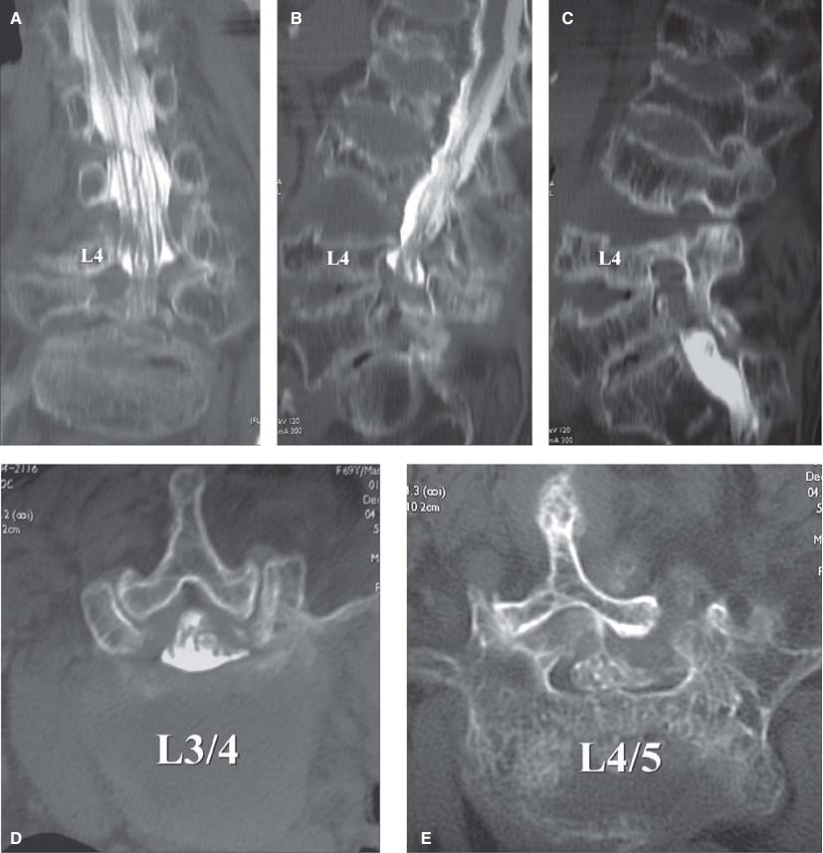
A 69-year-old women, with right L4 radiculopathy as revealed by postmyelographic CT scan. A: In the coronal section, bilateral L4 roots were well depicted. B: In the sagittal section, osteoporosis is evident; multiple vertebral collapse and end-plate irregularity are observed despite no history of trauma. C: In the sagittal section through the right foramen, foraminal stenosis is not obvious. D: In the transverse section (L3/4), spinal canal stenosis is very mild. E: In the transverse section (L4/5), prominent L4/5 facet joint destruction and lateral dislocation are noted.
